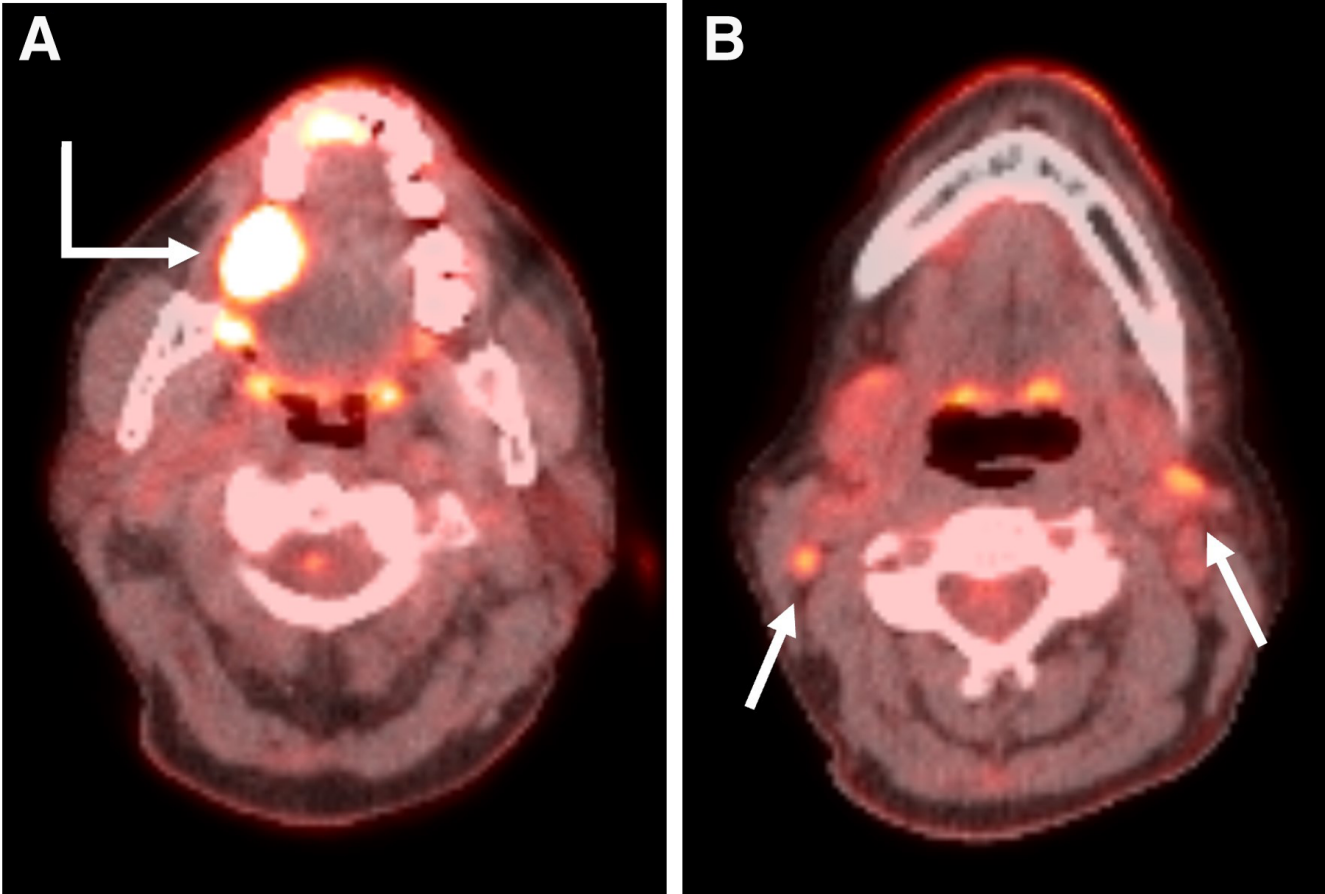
Axial PET/CT fusion images through the oral cavity (A) and neck (B) in a 59-year-old woman with a primary SCC in the right retromolar trigone (Bent arrow). Axial image through the oral cavity demonstrates the primary malignancy. Axial image through the neck demonstrates small bilateral level 2 hypermetabolic lymph nodes (Straight arrows). These were interpreted as suspicious for malignancy by the interpreting radiologist and the patient underwent bilateral neck dissections. No nodal metastases were present on surgical pathology.
